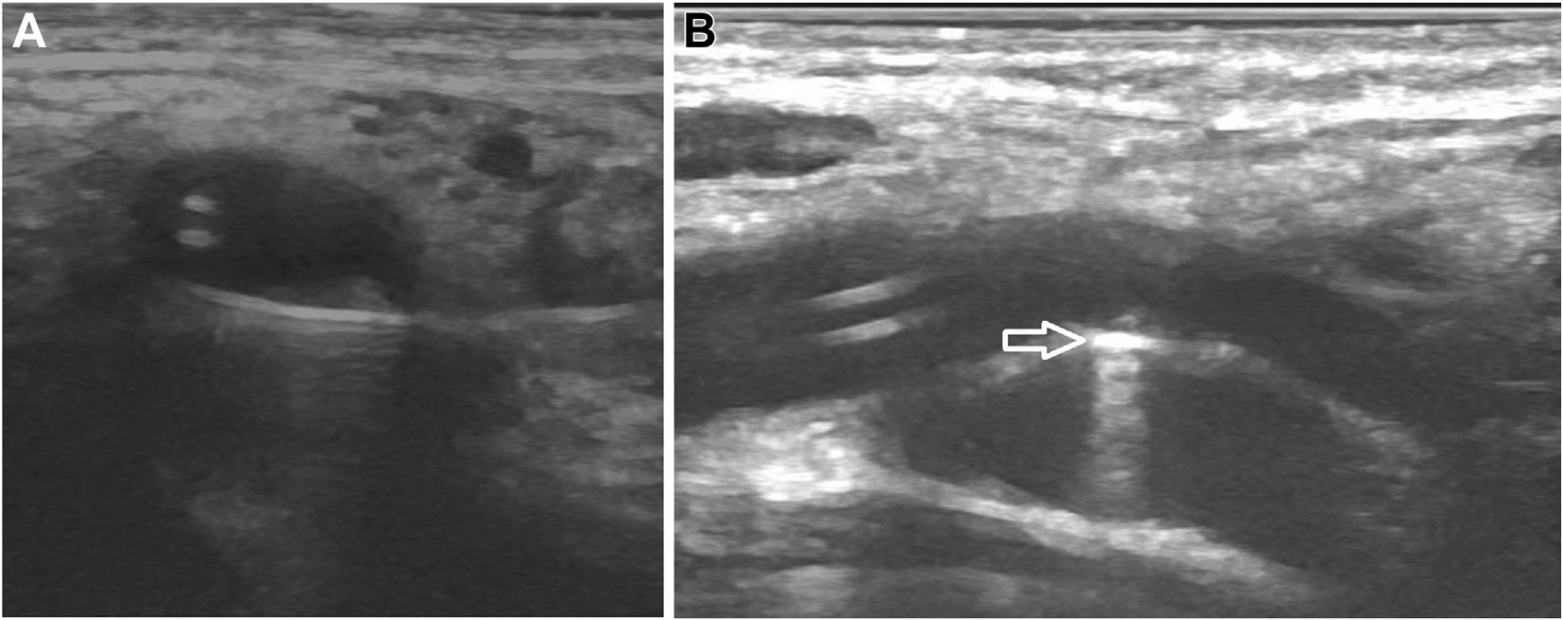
Sonographic-Guided Needle Placement (A) Transverse and (B) longitudinal ultrasound images demonstrating needle placement through the fistula (arrow).
